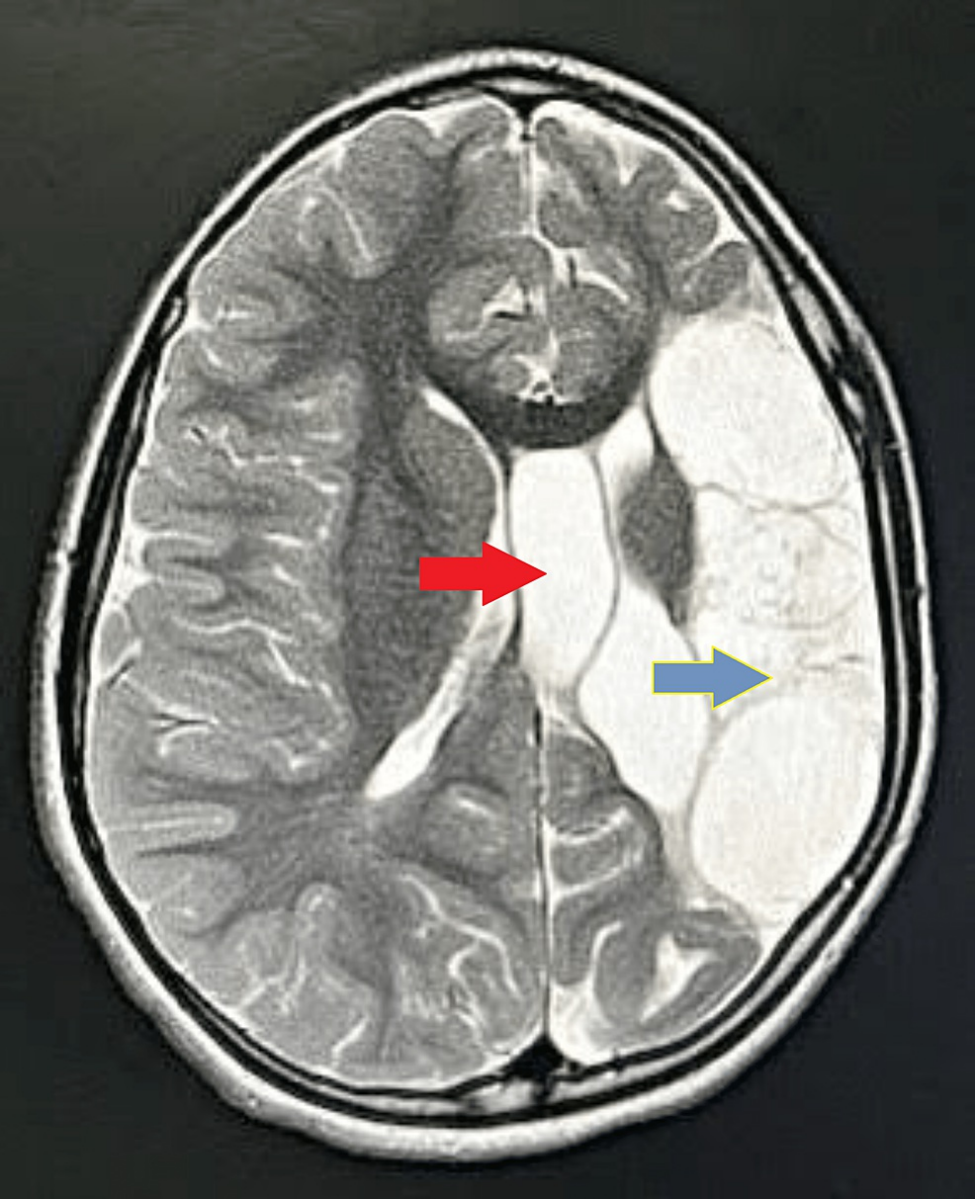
MRI brain axial T2WI Altered signal intensity is noted at the frontoparietal lobe on the left side with the loss of gray-white matter junction, encephalomalacia, and gliotic changes with dilatation of the anterior horn and body of ipsilateral lateral ventricle appearing hyperintense on T2WI Red arrow: dilated left lateral ventricle. Blue arrow: altered signal intensity at the left frontoparietal lobe MRI: magnetic resonance imaging; T2WI: T2 weighted imaging
